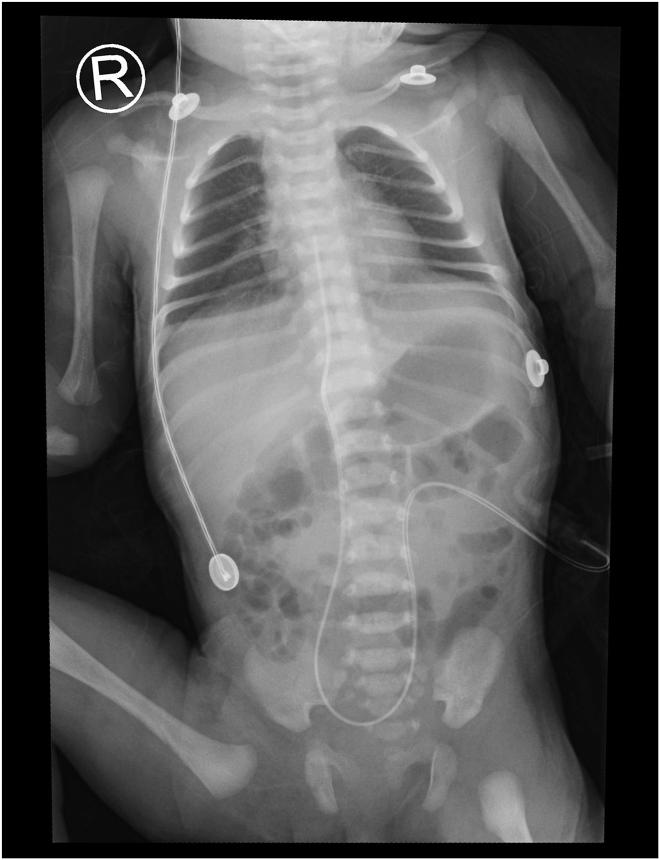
Neonatal pneumopericardium is exceedingly rare. This case is notable for the spontaneous occurrence of isolated pneumopericardium in a term neonate without antecedent trauma or identifiable risk factors beyond the initial respiratory distress. Crucially, the infant demonstrated rapid clinical stabilization and complete radiographic resolution with conservative management alone, avoiding invasive pericardial drainage. Based on rapid clinical improvement without tamponade signs, pericardiocentesis was deferred; it would be considered for hemodynamic compromise. Although idiopathic, a full workup includes echocardiography, computed tomography, and microbiology, but was not needed due to swift resolution.
